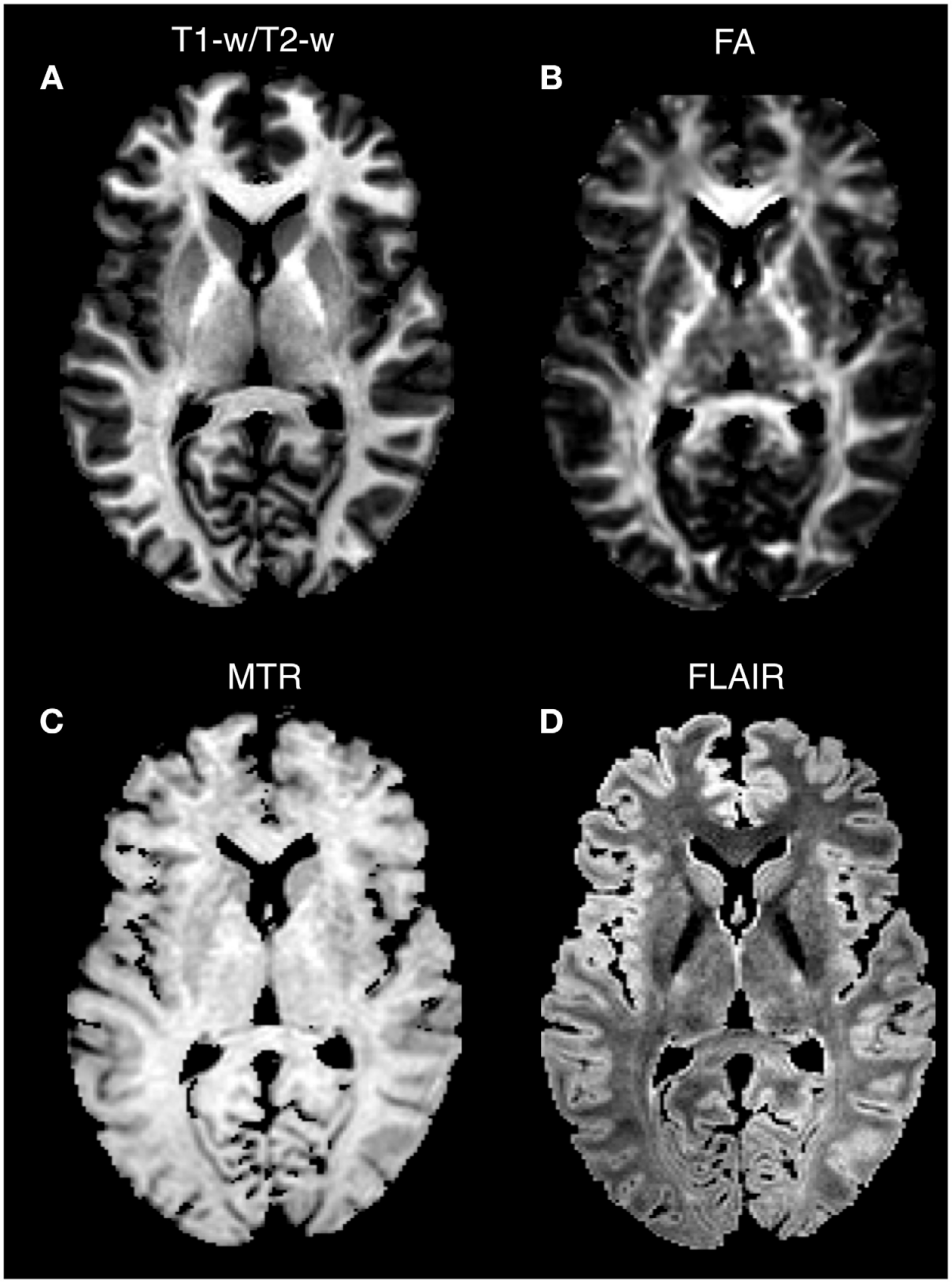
Single-subject images: comparison between image modalities. T1-w/T2-w (A), FA (B), MTR (C), and FLAIR (D) images for Subject 30 of the KIRBY database are shown in an axial section. Since conventional MRI images have arbitrary intensity scales, the four modalities are scaled according to the 1 and 99th percentiles. Note that higher intensity values in T1-w/T2-w, FA, and MTR characterize structures with a greater degree of myelination, whereas an inverted intensity scale defines the FLAIR technique.
